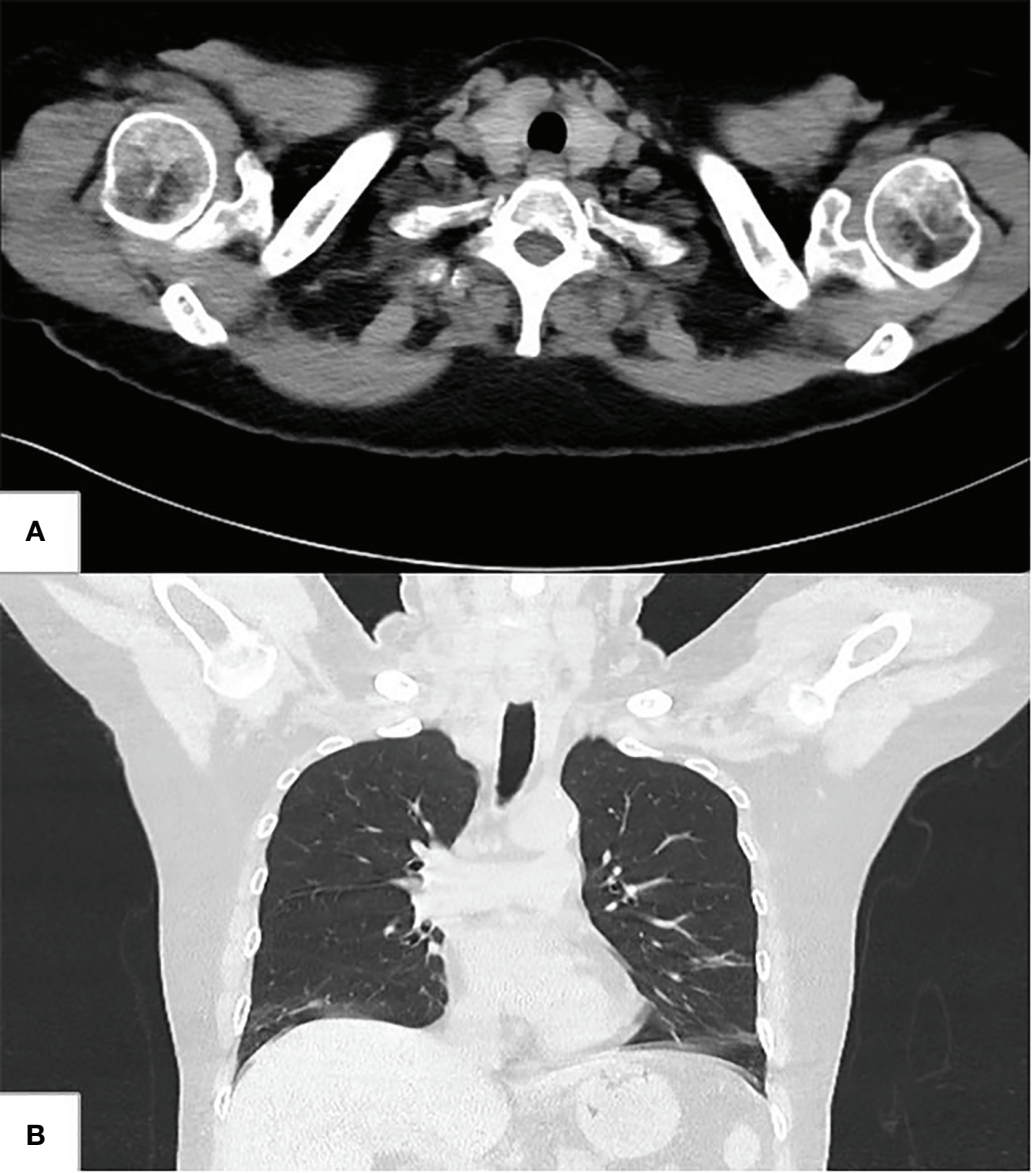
Postoperative neck–chest CT. (A) Transverse image shows the right anterior wall of the cervical trachea returned to normal. (B) coronal image shows the right anterior wall of the cervical trachea returned to normal.
